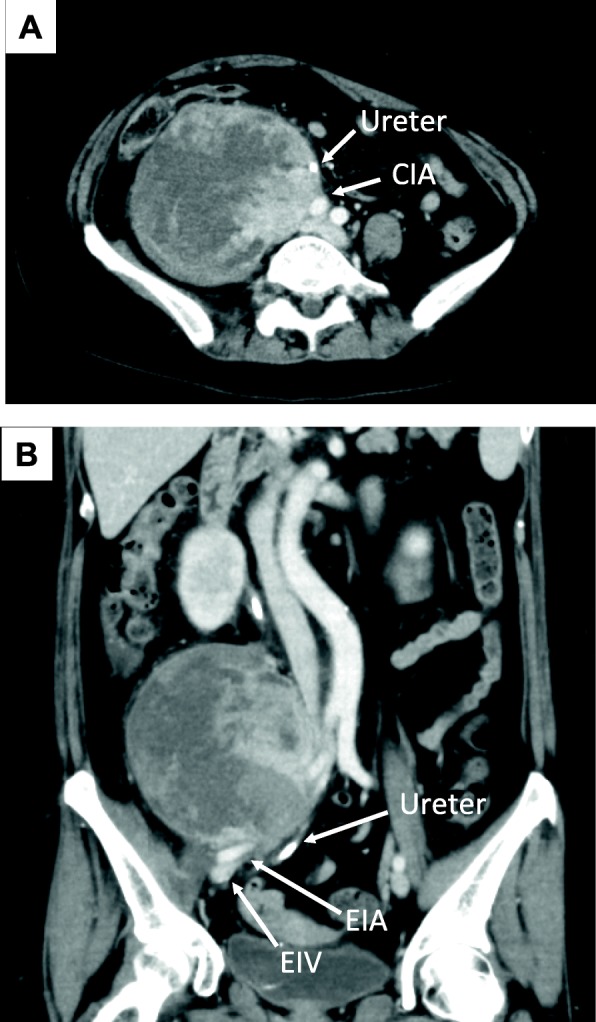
CT scan images of the retroperitoneal tumor. a Axial image. b Sagittal image after multiplanar reconstruction. CIA, common iliac artery; EIA, external iliac artery; EIV, external iliac vein
